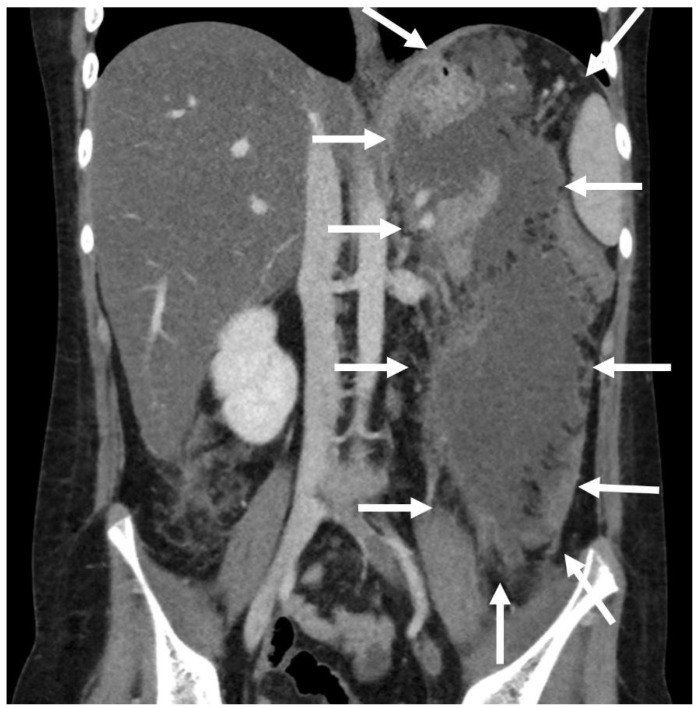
Patient n°3 CT. Necrotic-hemorrhagic pancreatitis (body/tail origin) with voluminous walled-off necrosis developing in the left quadrant (hypochondrium/flank; relations to stomach, spleen, kidney, descending colon), extending craniocaudal about 22 cm.
